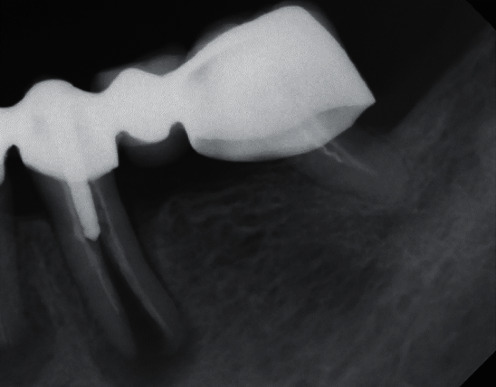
Radiograph tooth 35 at intake August 2018.
